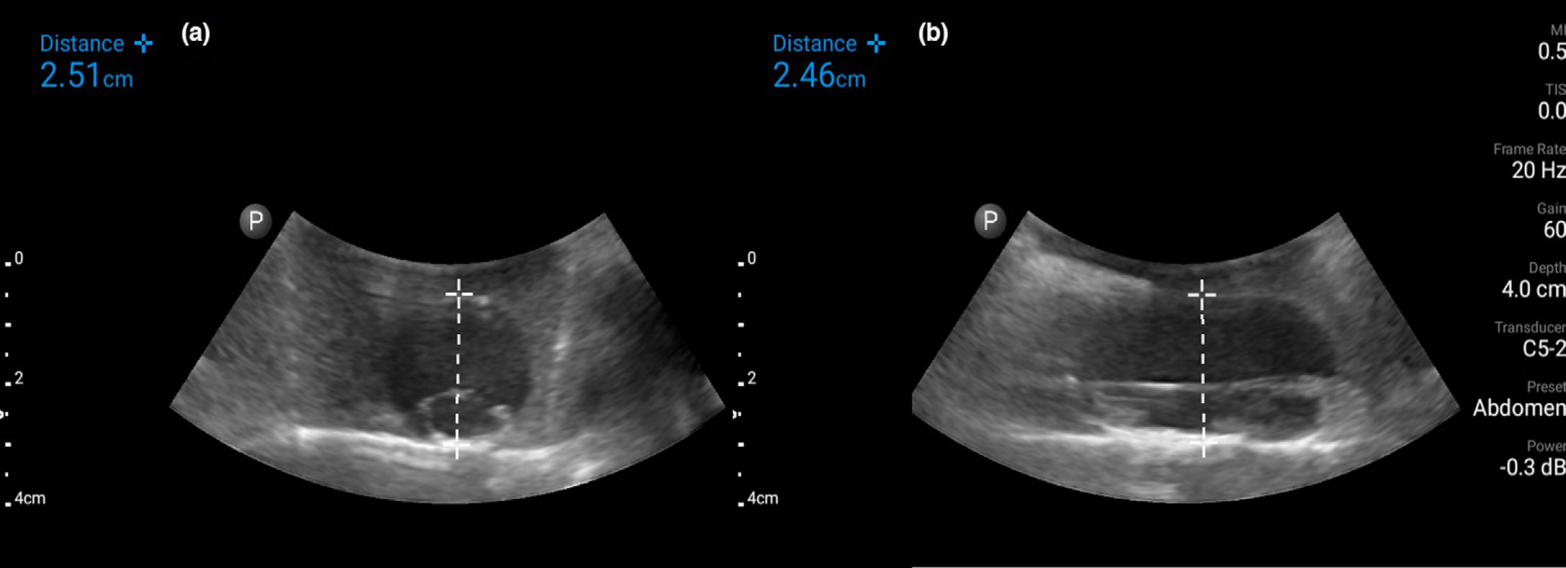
Ultrasound image using a low‐frequency curvilinear probe of the aortic dissection construct in gelatin mixture in transverse (a) and longitudinal (b) orientation with measurements. [Colour figure can be viewed at wileyonlinelibrary.com]
